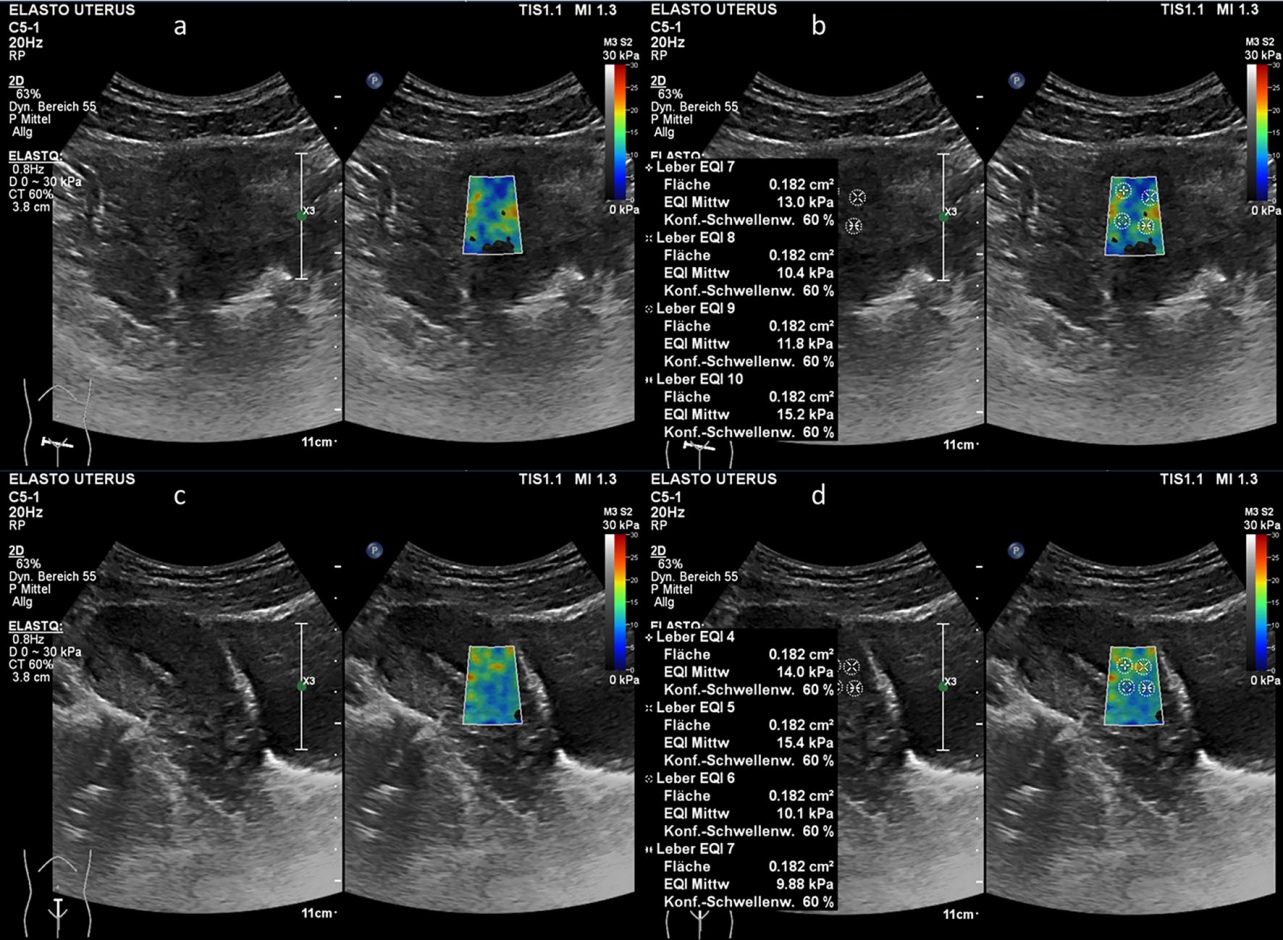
Transabdominal ultrasound examination including shear wave elastography (SWE) of the uterine myometrium (a) Transverse B-scan of the uterus (left side) and placement of the region of interest (ROI) on uterine myometrium for SWE measurements (right side) (b) Placement of sample points within the ROI on the transverse B-scan to measure tissue stiffness (c) Longitudinal B-scan of the uterus (left side) and placement of the ROI on the uterine myometrium for SWE measurements (right side) (d) Placement of sample points within the ROI on the longitudinal scan to measure tissue stiffness.
